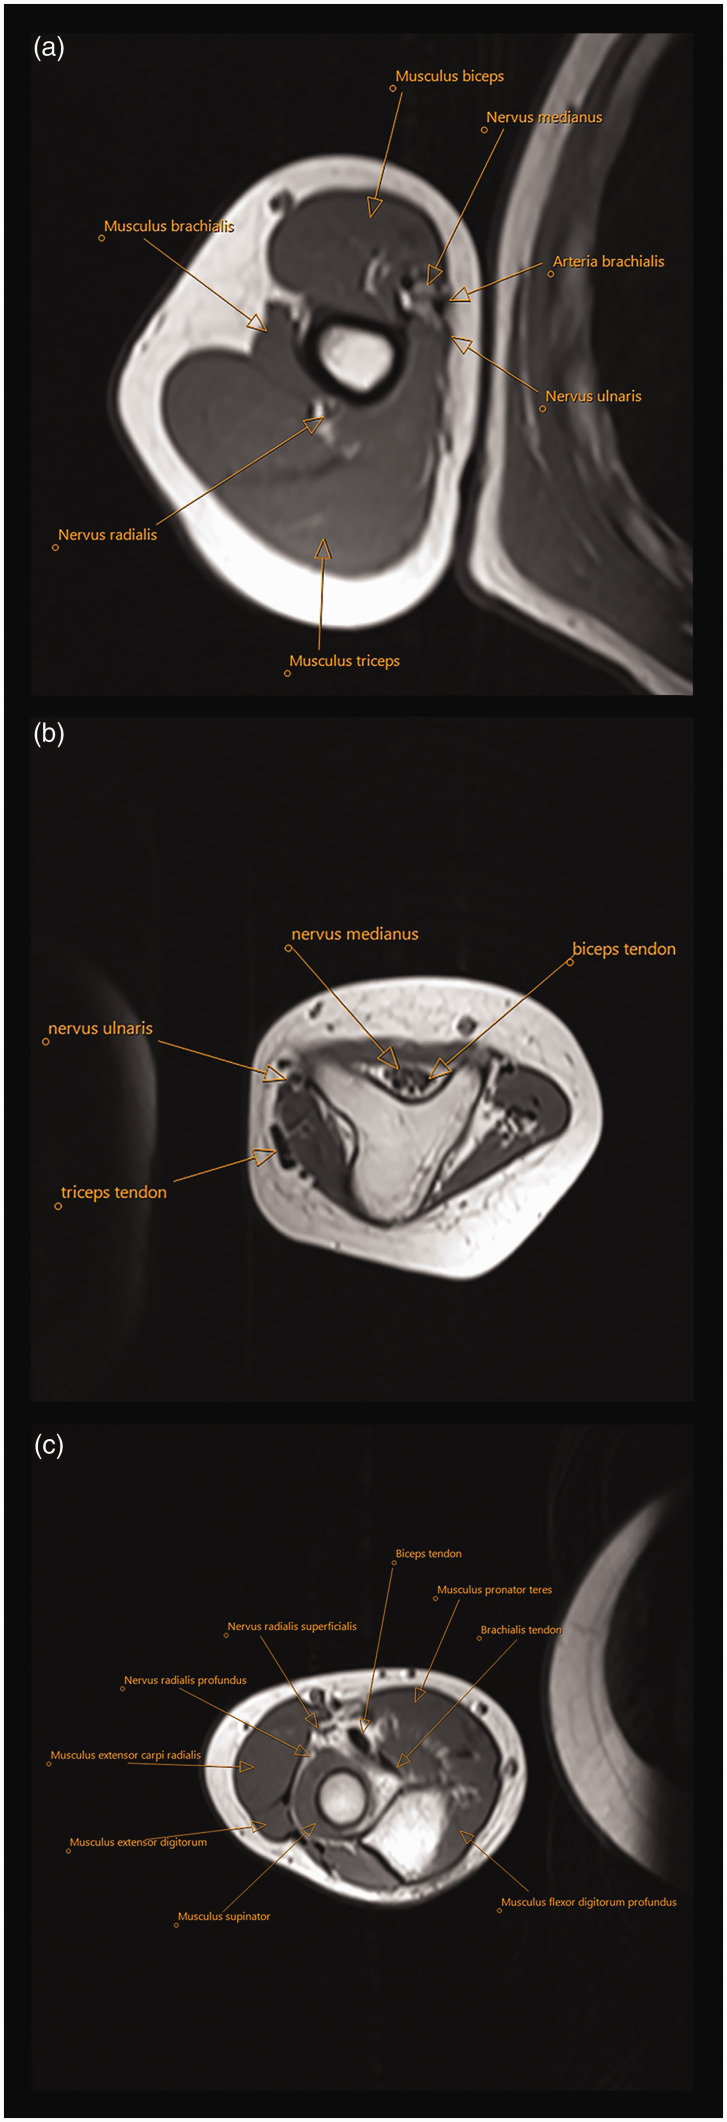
Case 9. MRI sections (a) at mid-upper arm, (b) of the triangular shaped dysplastic distal humerus and (c) just distal to the elbow joint, depicting two proximal ulnae. Identification of anatomical structures of interest such as biceps, brachialis and triceps muscles is possible.
